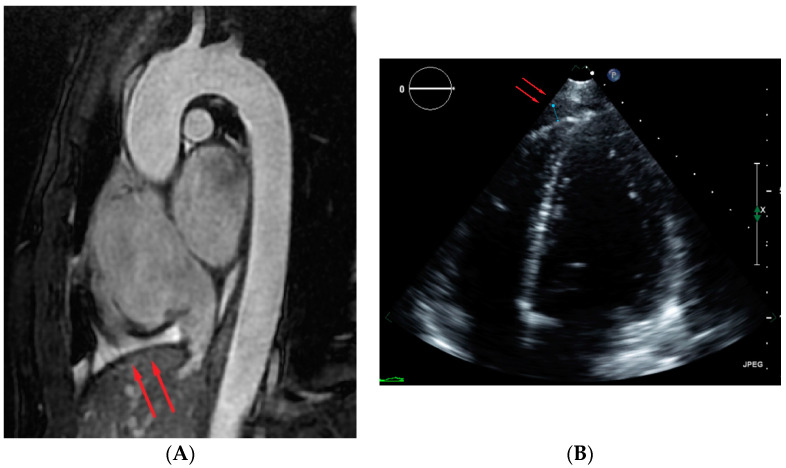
Asymmetrically distributed pericardial effusion (red arrows) on (A) MRI and (B) echocardiography.
